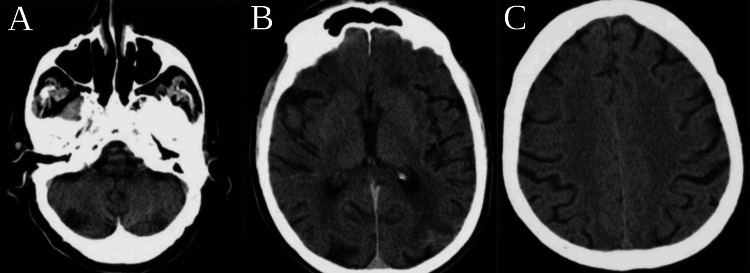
Computed tomography scan of the brain A: axial CT scan at the infratentorial level; B: axial CT scan at the supratentorial level passing through the ventricles; C: axial CT scan at the supratentorial level passing through the frontoparietal lobes.
